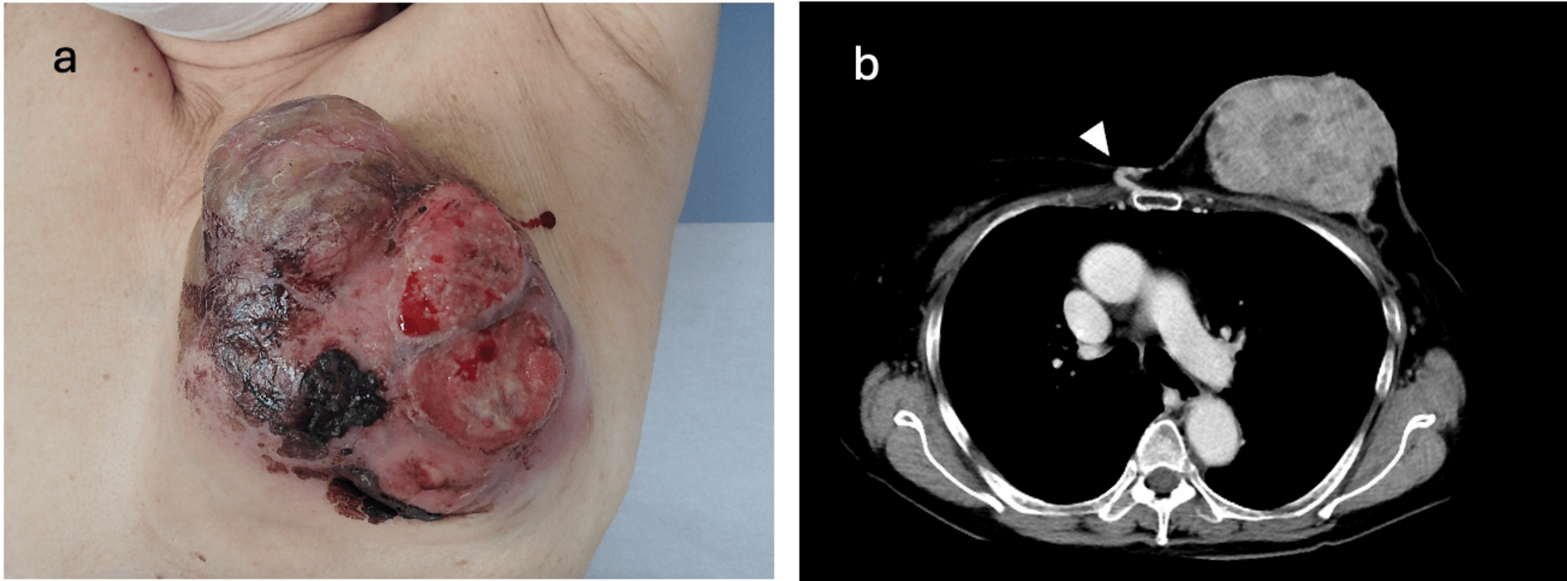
(a) Patient photograph taken before the cardiopulmonary arrest (CPA). (b) Contrast-enhanced CT scan showing a 600 × 100 mm mass. The arrowhead indicates the left internal mammary artery.
